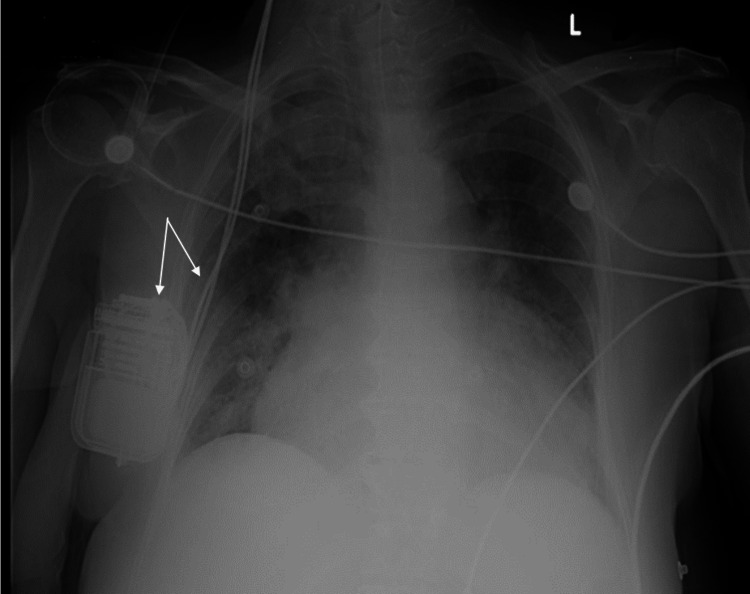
AP chest X-ray The image shows bilateral lower lobe infiltrates, more profound on the right, and cardiomegaly; the DBS device and leads are visible (white arrows). AP: Anteroposterior; DBS: Deep Brain Stimulation
